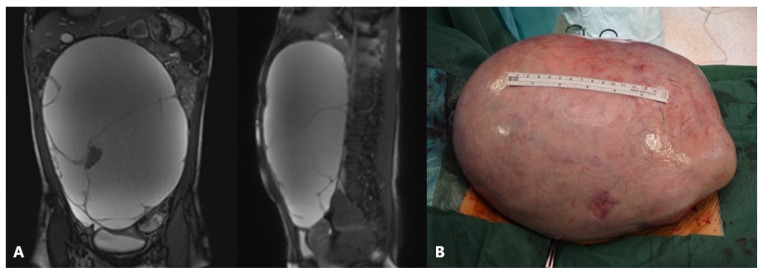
A 14-year-old girl presented with abdominal distension that had been present for several months. Apart from occasional constipation, she had no other symptoms. (A) MRI of the abdomen shows an intraperitoneal mass inseparable from the right ovary, compressing the pancreas and abdominal aorta; (B) Surgical exploration of the abdomen—mature cystic ovarian teratoma with foci of adenocarcinomas measuring 28 × 22 × 14 cm. Source: Archive of the Department of Pediatric Surgery, Children’s Hospital Zagreb.
